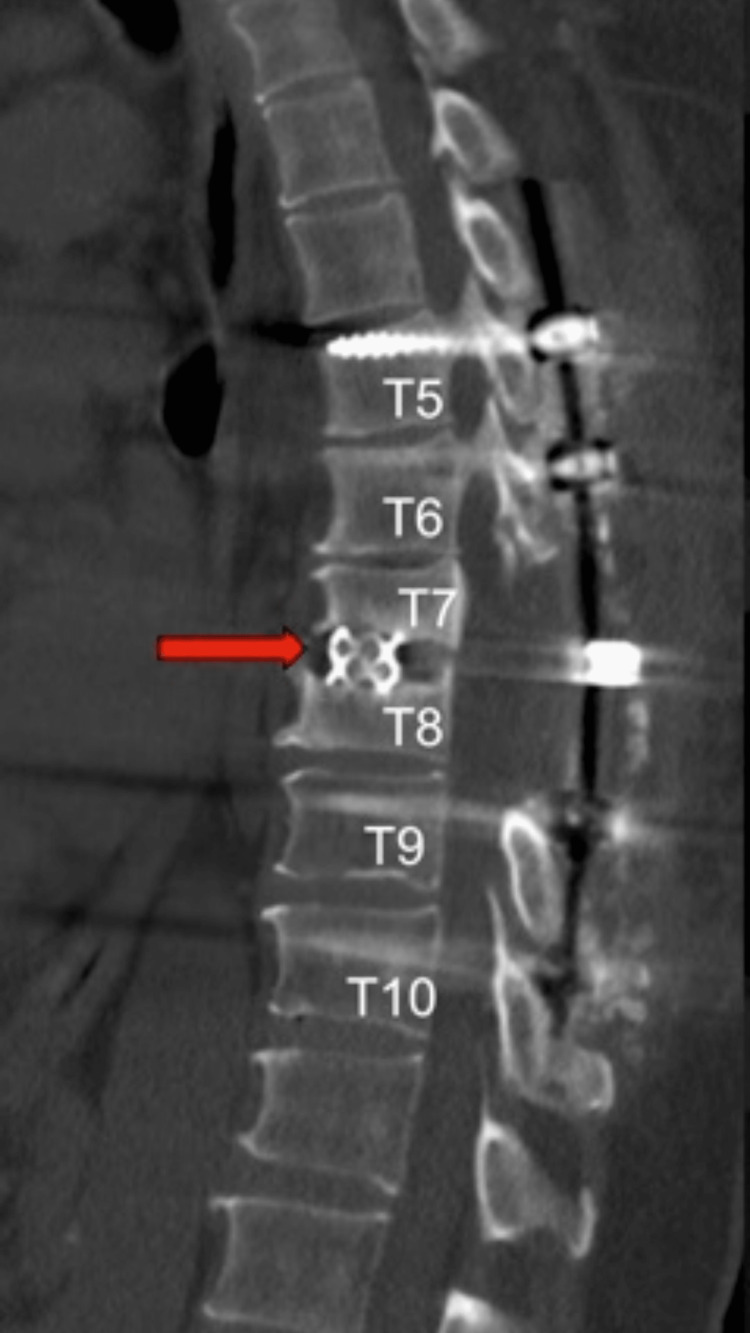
T1-weighted sagittal view after right costotransversectomy and partial anterior corpectomies at T7 and T8 with T7-T8 anterior fusion (red arrow) and posterior T5-T10 fusion.
